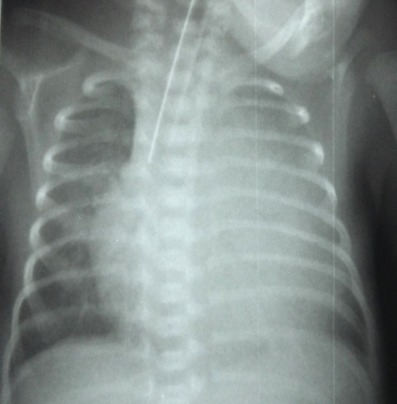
Chest x-ray showing increase of the cardiac area
